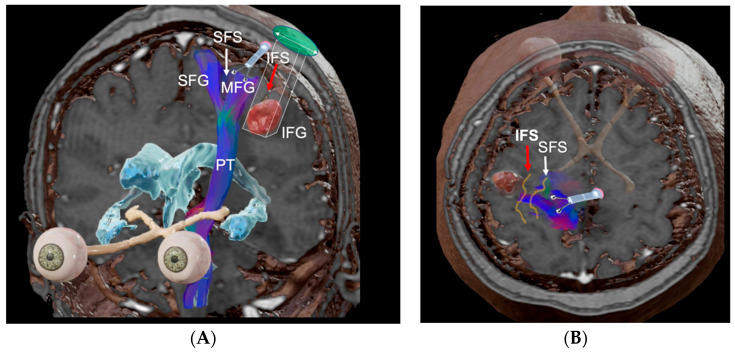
An intra-axial tumor at the junction of the inferior frontal gyrus and the pre-central sulcus. Fig (A) shows coronal view of merged T1 weighted MRI scan with corticospinal tract tractography. Fig (B) shows the axial view of the latter.
